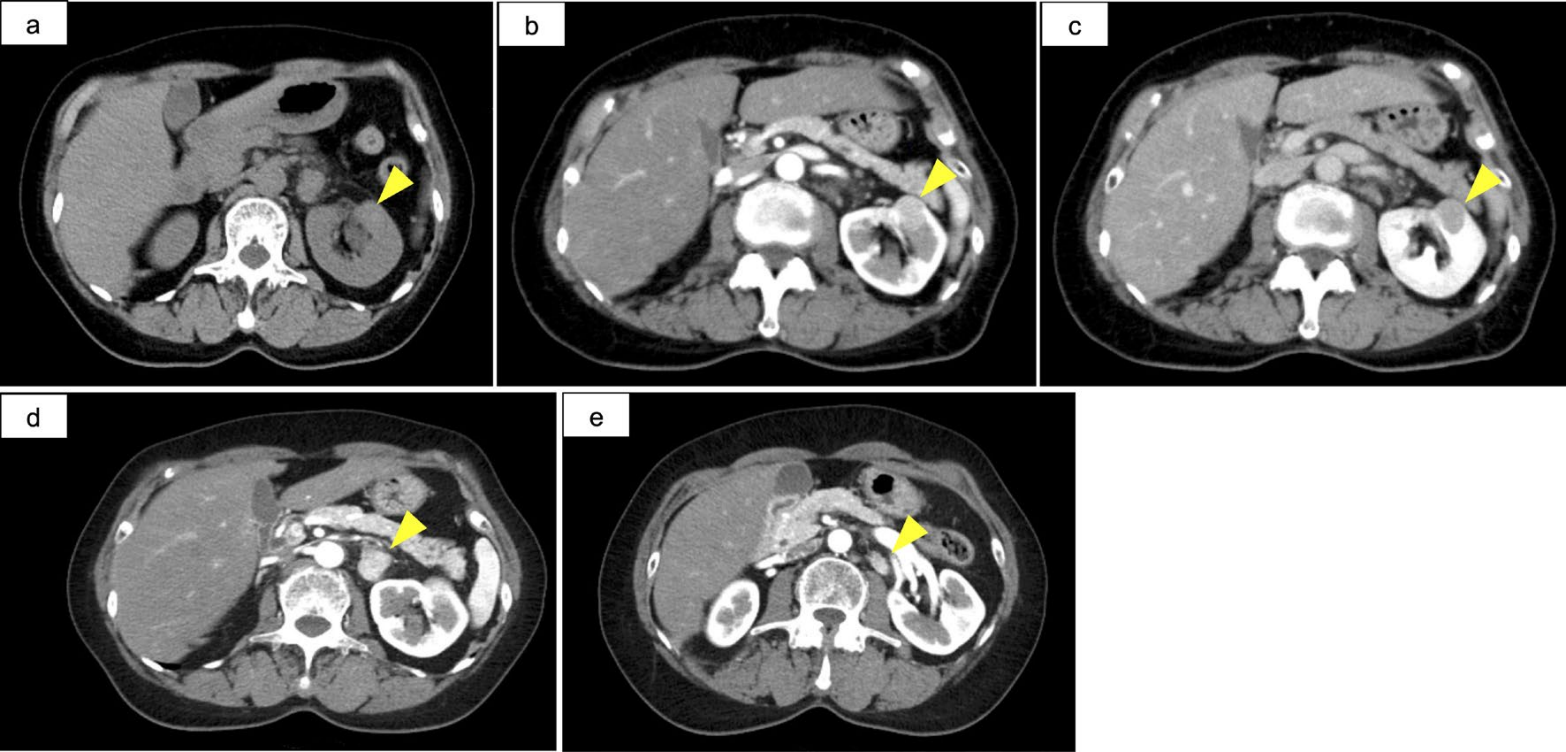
Preoperative contrast‐enhanced CT images. (a) Non‐contrast image. (b) Early‐phase contrast‐enhanced CT showing relatively homogeneous enhancement of the renal tumor. (c) Late‐phase contrast‐enhanced CT showing gradual washout of the renal tumor. (d, e) Early‐phase contrast‐enhanced CT showing two enlarged lymph nodes in the renal hilar region with early enhancement.
